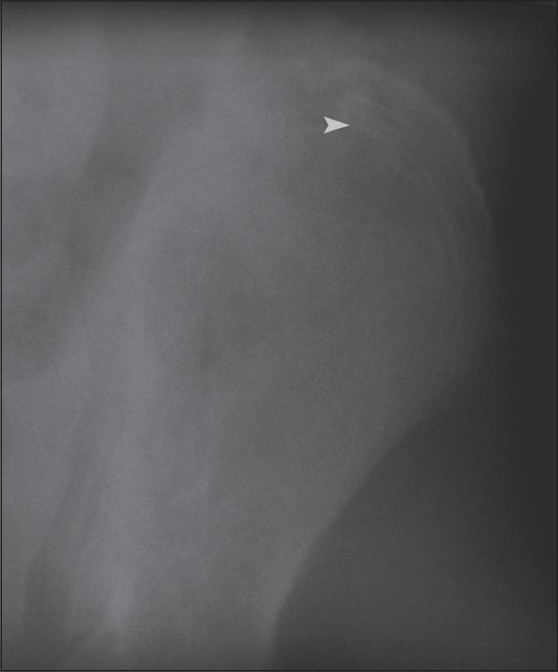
Frontal radiograph of the pelvic bones shows zebra lines involving the iliac crest (white arrow head)
